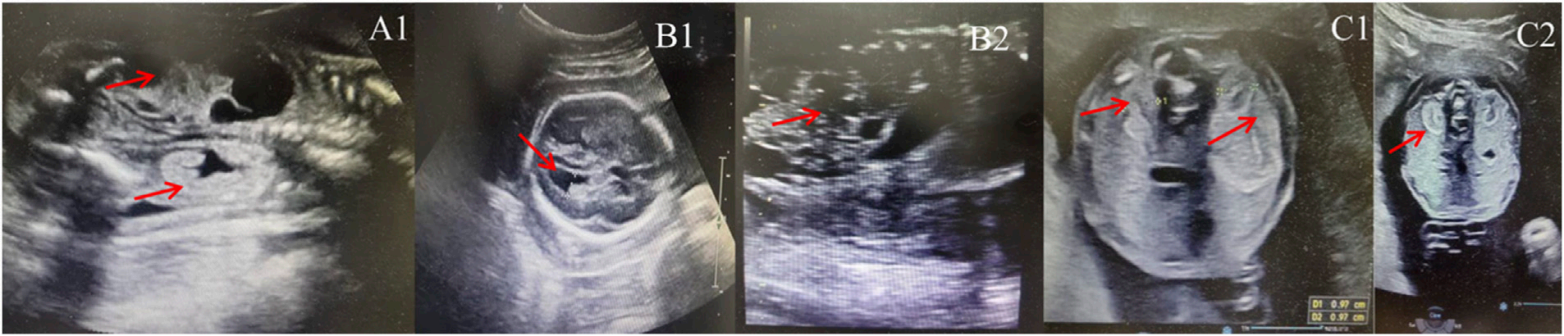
Sonographic characteristics of renal anomalies in fetuses with 17q12 deletion syndrome. (A1): left multicystic dysplastic kidney and right hyperechogenic kidney of fetus case 1; (B1): increased width of the posterior corner of the lateral ventricle of fetus 2 (10 mm); (B2): left multicystic dysplastic kidney of fetus case 2; (C1): Horizontal gray ultrasound image of both kidneys demonstrates smaller kidney sizes with 1.0 cm of fetus case 3; (C2): bilateral hyperechogenic kidneys of fetus case 3.
